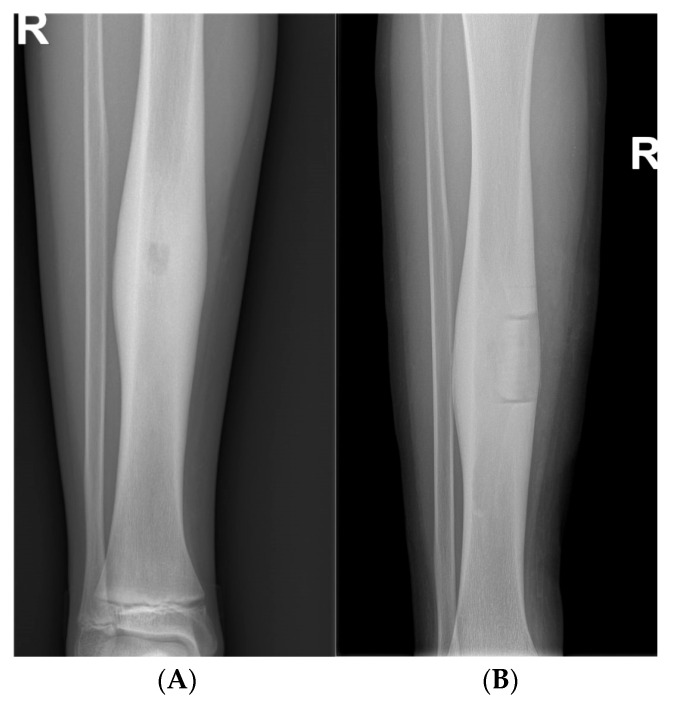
X-ray images of a 13-year-old patient with right tibial osteoid osteoma before (A) and after (B) surgery. The preoperative image shows a well-defined nidus. The postoperative radiograph demonstrates the appearance following fenestration, biopsy, curettage.
